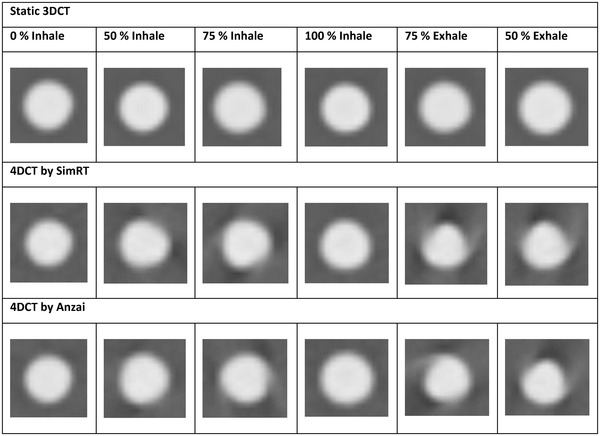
Note*: A tumor target with a diameter of 1 cm was used. This table represents the upper tumor trajectory A depicted in Figure 6. Abbreviations: CT, computed tomography; 3D, 3‐dimensional; 4D, 4‐dimensional.
